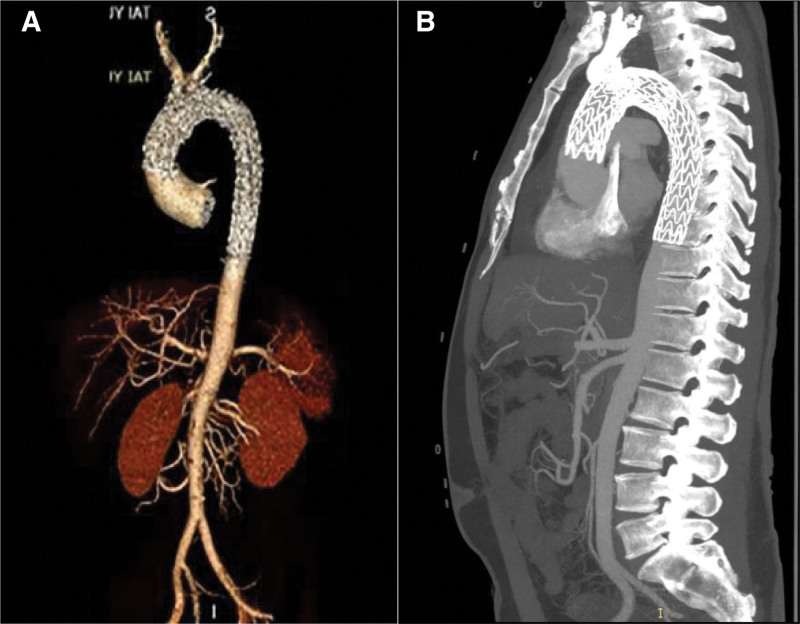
The images of the 3-mo follow-up. CTA showing that the aortic remodeling was good, and there was no stent graft-induced redissection. CTA = computed tomography angiography.
